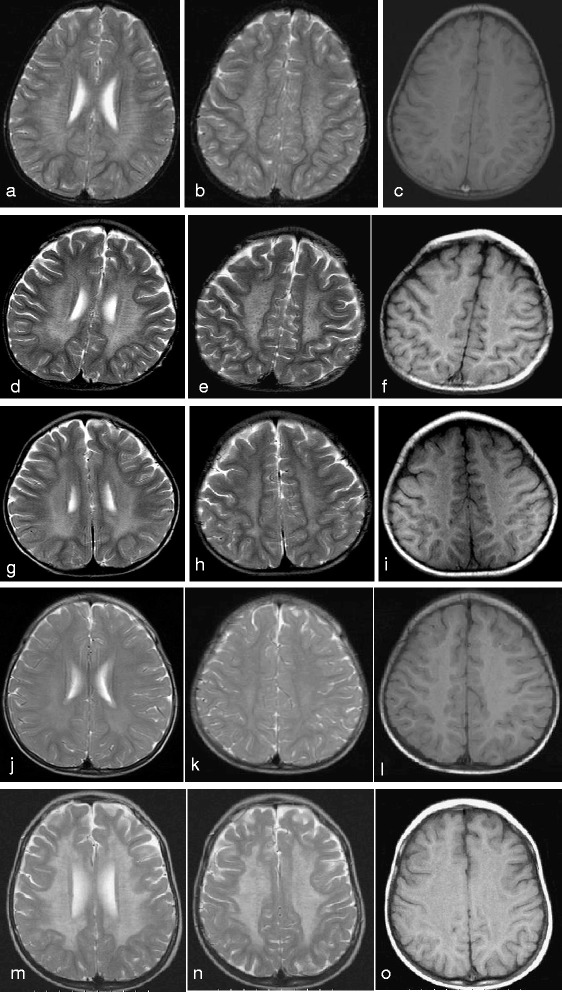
Cranial MRIs of our patients. a-c represents patient 1, d-f patient 2, g-i patient 3, j-l patient 4, and m-o patient 5. (a, d, g, j, m) Hypointense radially oriented stripes and dots seen within the hyperintense cerebral white matter (resembling tiger skin) on T2-weighted axial imaging. (b, e, h, k, n) Hypointense dots resembling leopard skin seen on T2-weighted axial imaging at the level of centrum ovale. (c, f, i, l, o) Iso to hyperintense dots seen in the cerebral white matter on T1-weighted imaging. This pattern of dysmyelination resembles the skin of tiger (radial stripes) and leopard (dots), the so-called tigroid and leopard pattern of dysmyelination in metachromatic leukodystrophy
